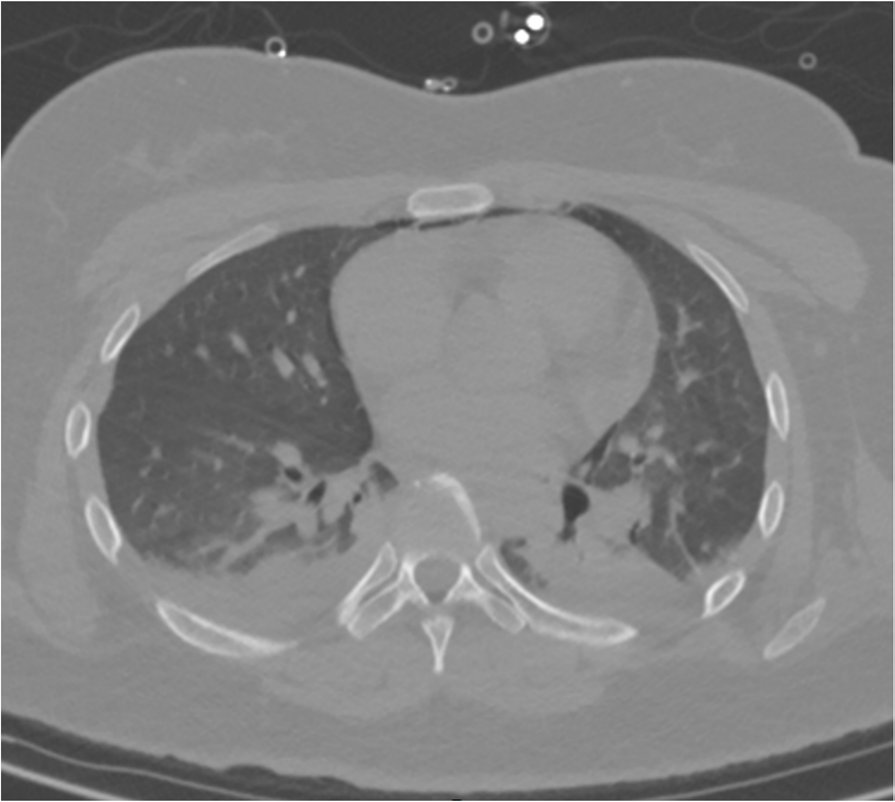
Postoperative chest computed tomography showing aspiration pneumonia
